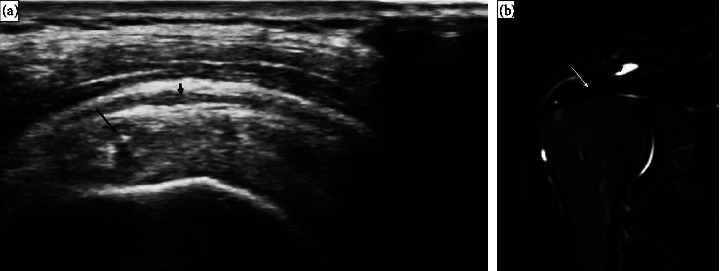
Ultrasound and MRI findings in a 46-year-old male patient with a 5-year history of hemodialysis with unilateral shoulder pain for 6 month. (a) US scan of supraspinatus tendon on its long axis shows thickness of the tendon with loss of its normal fibrillar pattern (long arrow) with minimal fluid collection at the subacromion subdeltoid bursa (short arrow) denoting supraspinatus tendinosis and subacromion subdeltoid bursitis. (b) MRI shoulder coronal STIR images revealed subacromion subdeltoid bursitis (short arrow) and supraspinatus tendinosis (long arrow).
